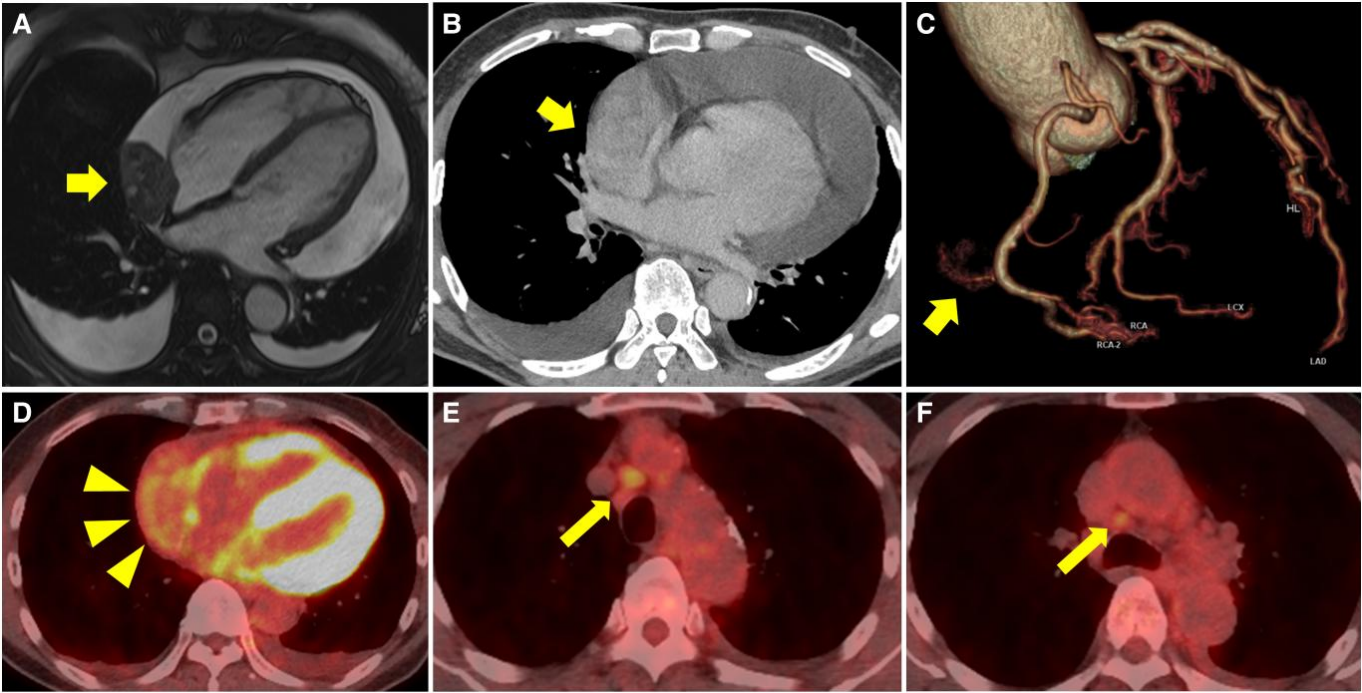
(*A* and *B*) Contrast-enhanced computed tomography (CT) and cardiac magnetic resonance imaging showed the mass, indicated by arrows and (*C*) coronary CT showed small arteries supplying the mass, also marked with a arrow, originating from the right coronary artery. (*D*) Positron emission tomography-CT showed increased uptake on the pericardial mass, indicated by arrowheads and (E and *F*) mediastinal lymph nodes, highlighted by long arrows.
